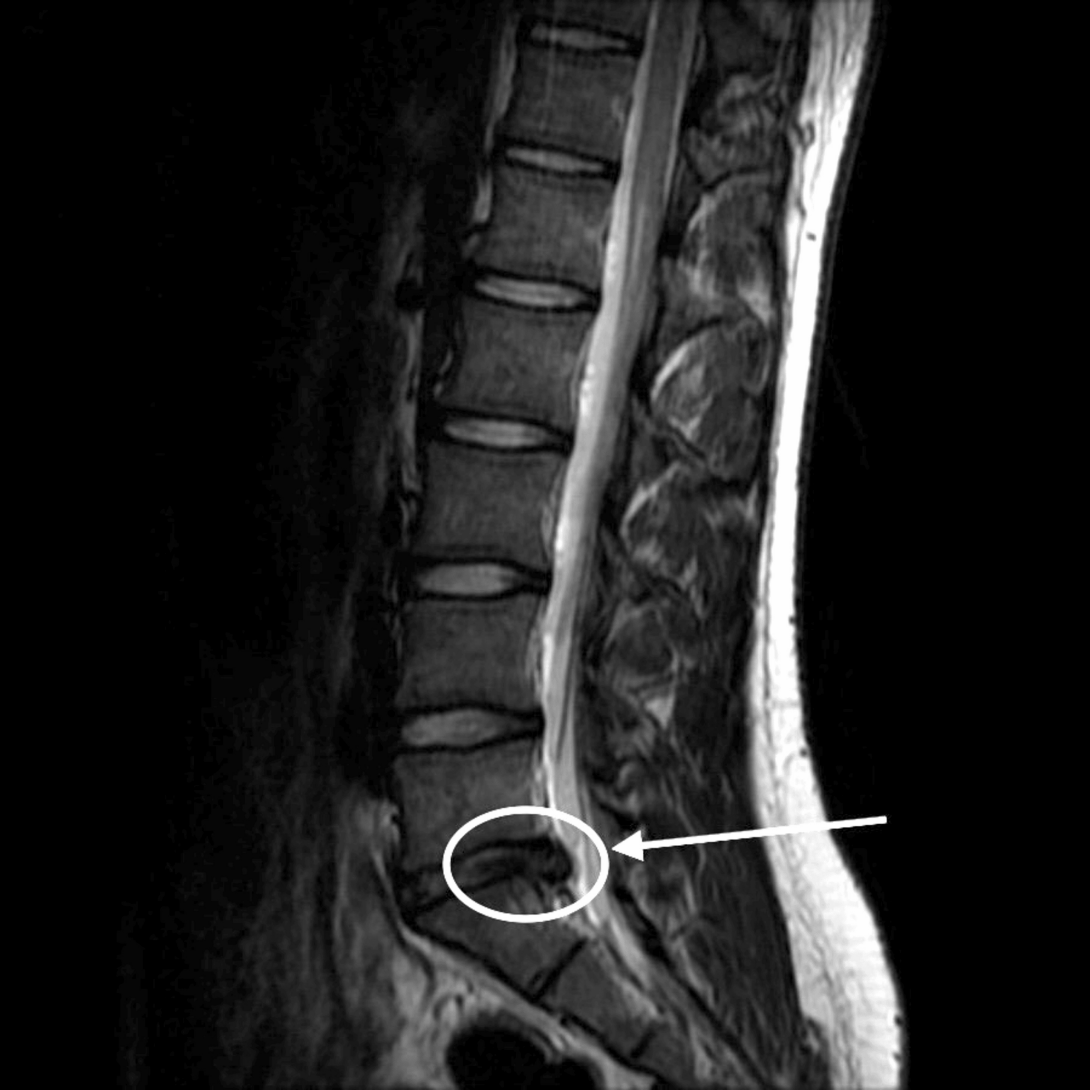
Sagittal T2-weighted MRI of the lumbar spine with a lateral herniation compressing the left L4 nerve root and a central-to-right paracentral herniation impacting the right S1 nerve root. There is a loss of lumbar lordosis (marked by circle).
